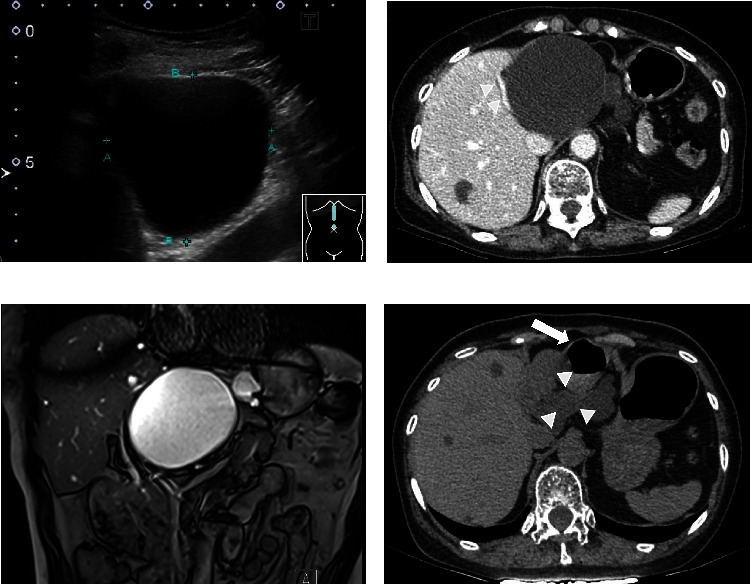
Ultrasonography revealed a giant cyst in the left lobe of the liver (a). The dimensions were 76 × 81 mm. No septa or mural nodules were observed. Contrast-enhanced computed tomography (CT) revealed that the liver cyst had replaced the left lobe. The left branch of the portal vein surrounded the hepatic side of the liver cyst (b) (arrowheads). Magnetic resonance cholangiopancreatography showed that the liver cyst had no communication with the bile duct. There were no neoplastic findings indicating a simple liver cyst (c). On the CT scan after the test puncture (d), the gastric antrum (arrow) migrated to the ventral side of the liver cyst (arrowheads).
